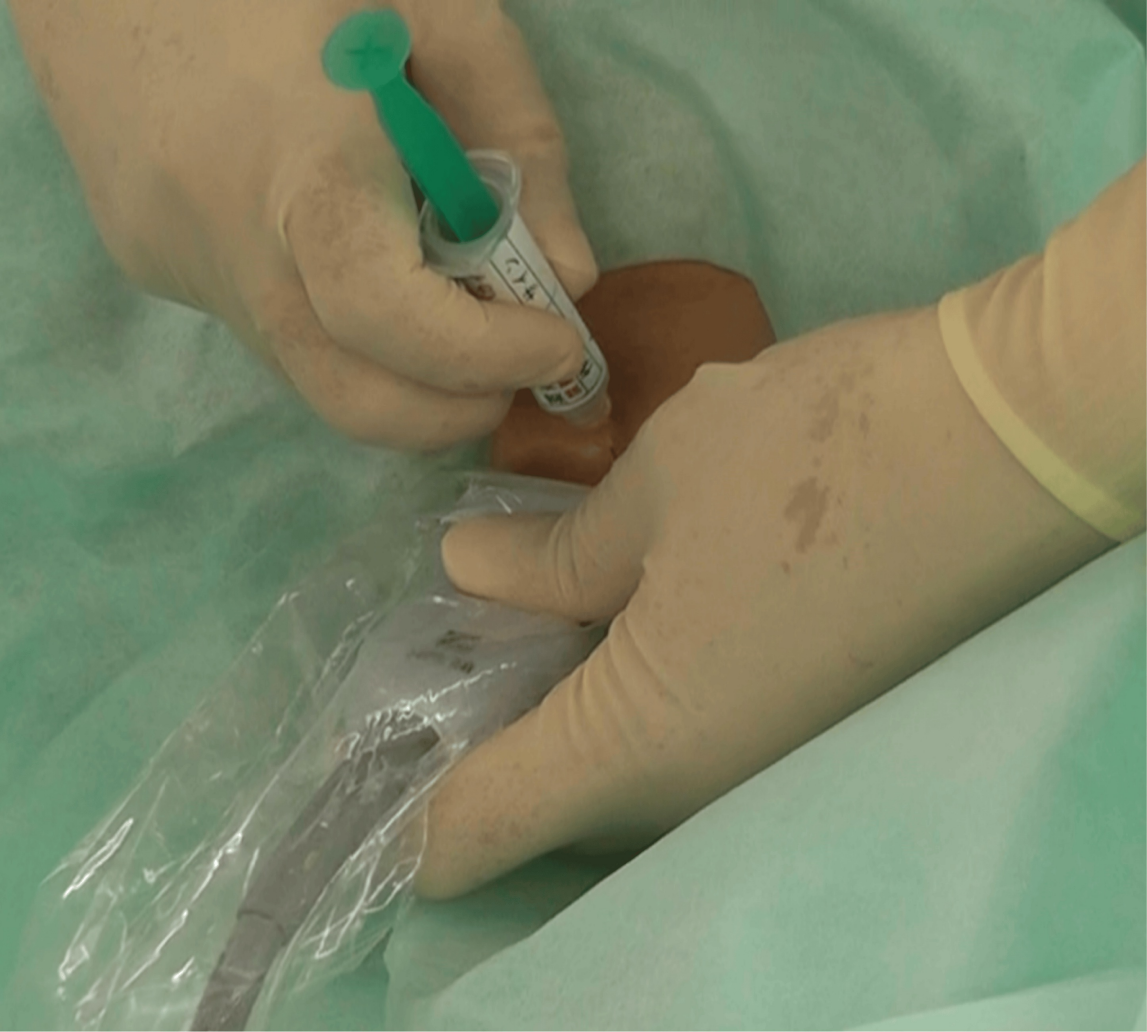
Ultrasound-guided selective glossopharyngeal nerve block to suppress the gagging reflex To perform intubation while maintaining spontaneous breathing with the three nerve blocks technique (3N technique), we first performed an ultrasound-guided selective glossopharyngeal nerve block. Reflex suppression was achieved after the block was performed.
